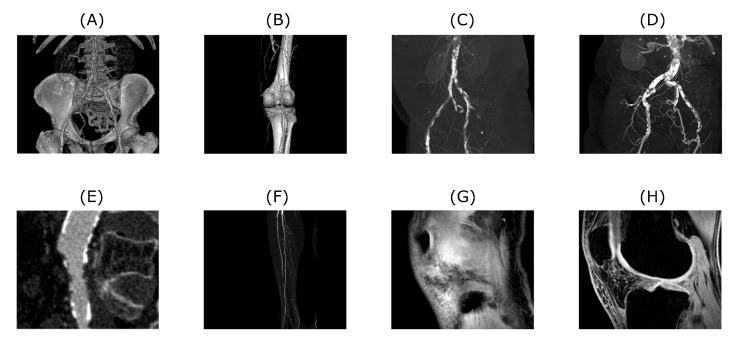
Examples of CT (A–F) and MRI (G and H) scans used to test the proposed algorithm. Tested images are showing various level of magnification and amount of detail. Also, native noise levels contained within these images are different. Images (A–F) are depicting blood vessel calcification in different details and perspectives, whereas images (G–H) are showing successive slices of knee cartilage.
